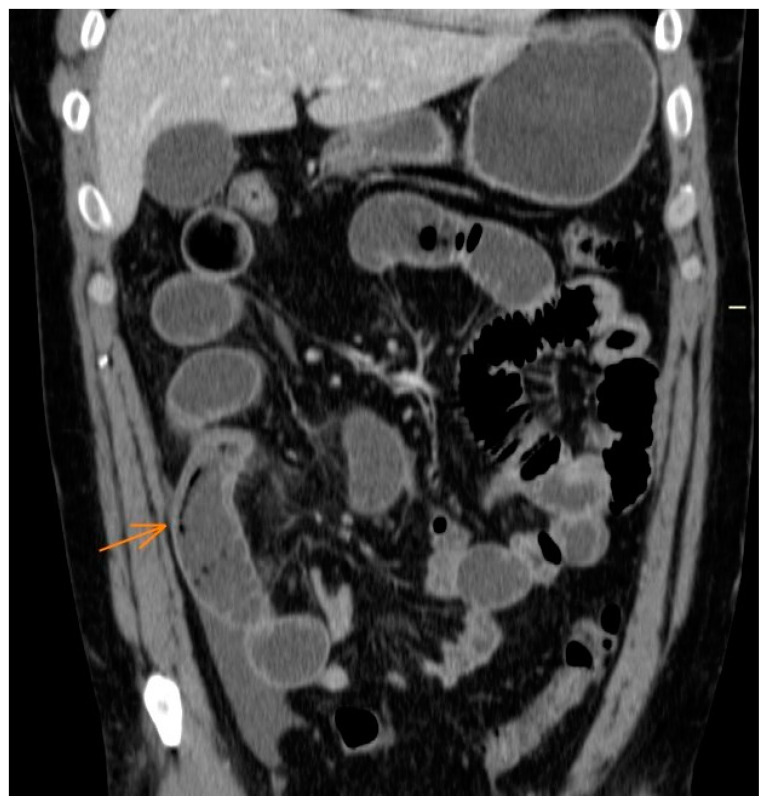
Abdominal CT: linear hypodense images in the lumen of the distal ileal loop in relation to the parasite. The arrows represent asymmetric mural thickening of the intestinal wall with submucosal edema.
